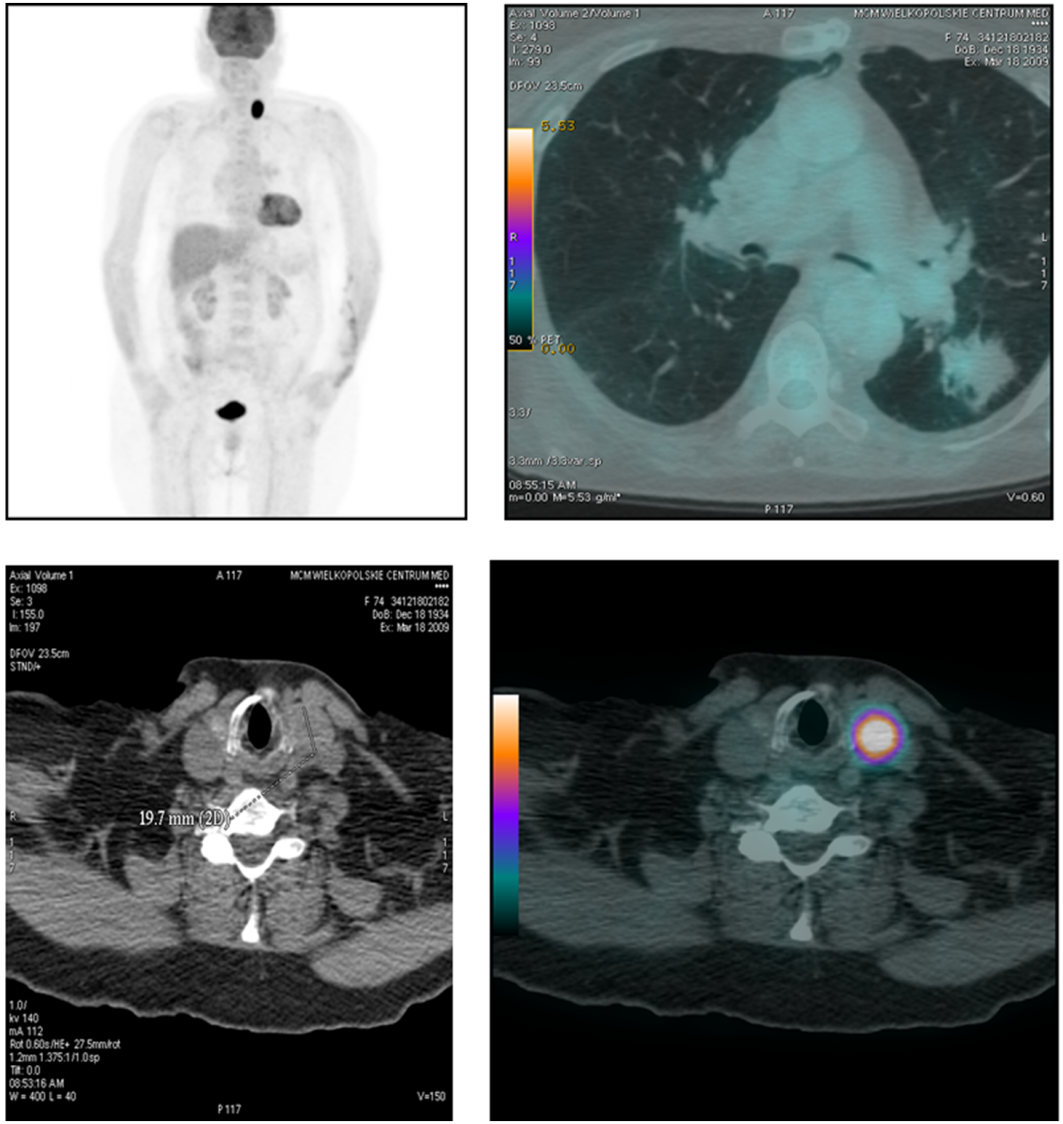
PET/CT scan performed in a female patient (74 years old) with a pulmonary tumor of indeterminant character. Incidental focal 18FDG uptake revealed in the left lobe of the thyroid gland (SUV_max_ 1.5). Colloid nodule in post-FNAB cytological evaluation.
